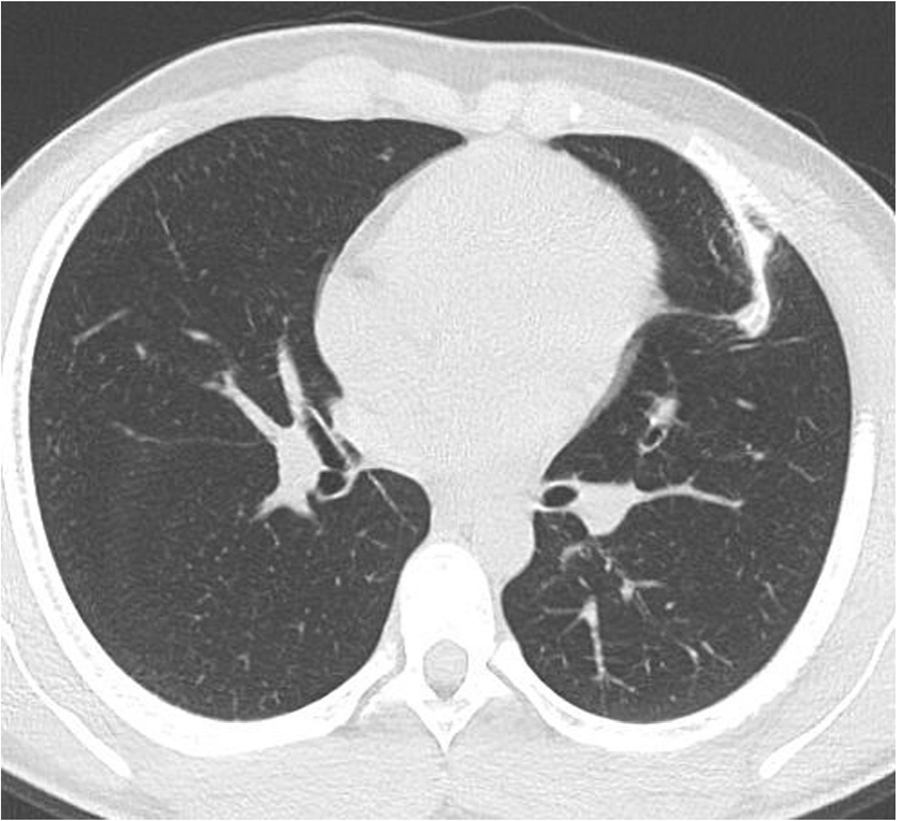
Chest computed tomography scan shows a rod-like mass with a soft tissue shadow adjacent to the left fifth rib
